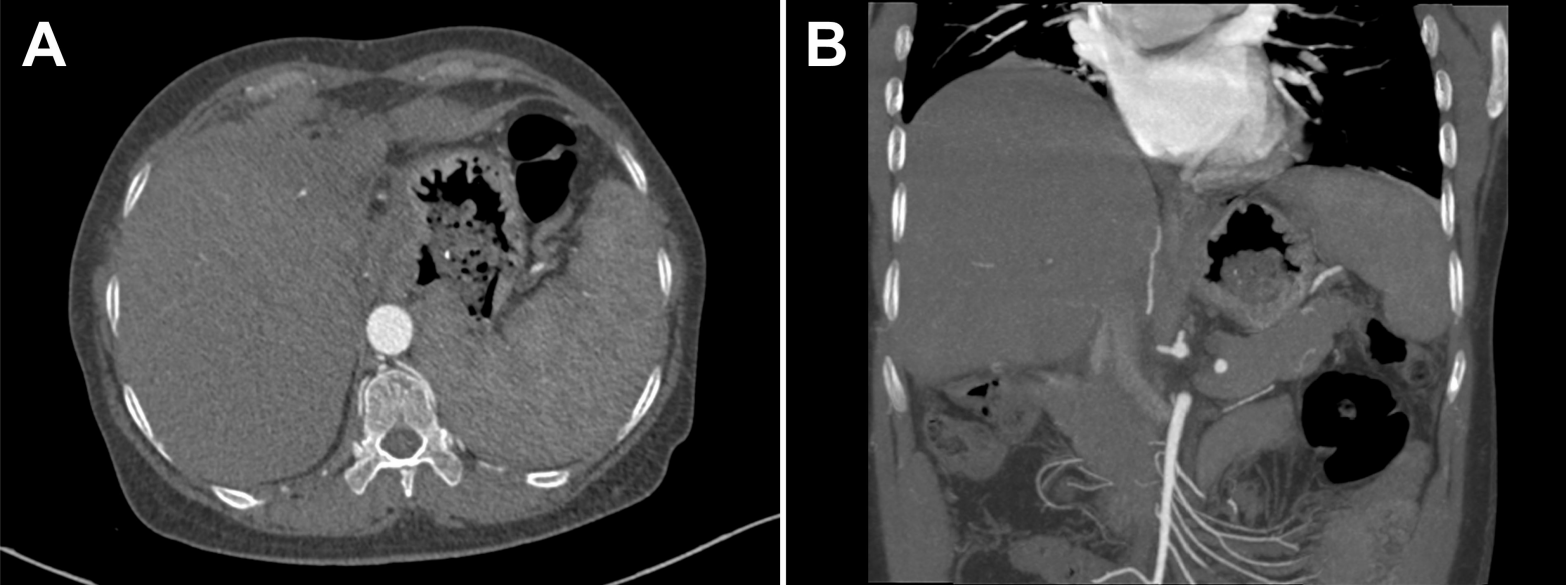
(A, B) Abdominal contrast-enhanced computed tomography (CT) showed the atrophic left hepatic lobe and enlarged spleen.
